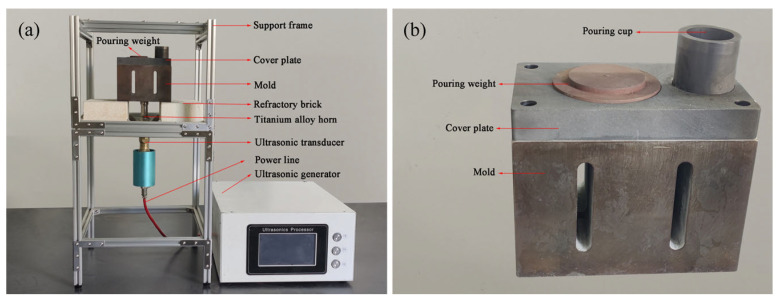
Ultrasonic vibration system: (a) experimental setup; (b) metal mold.
